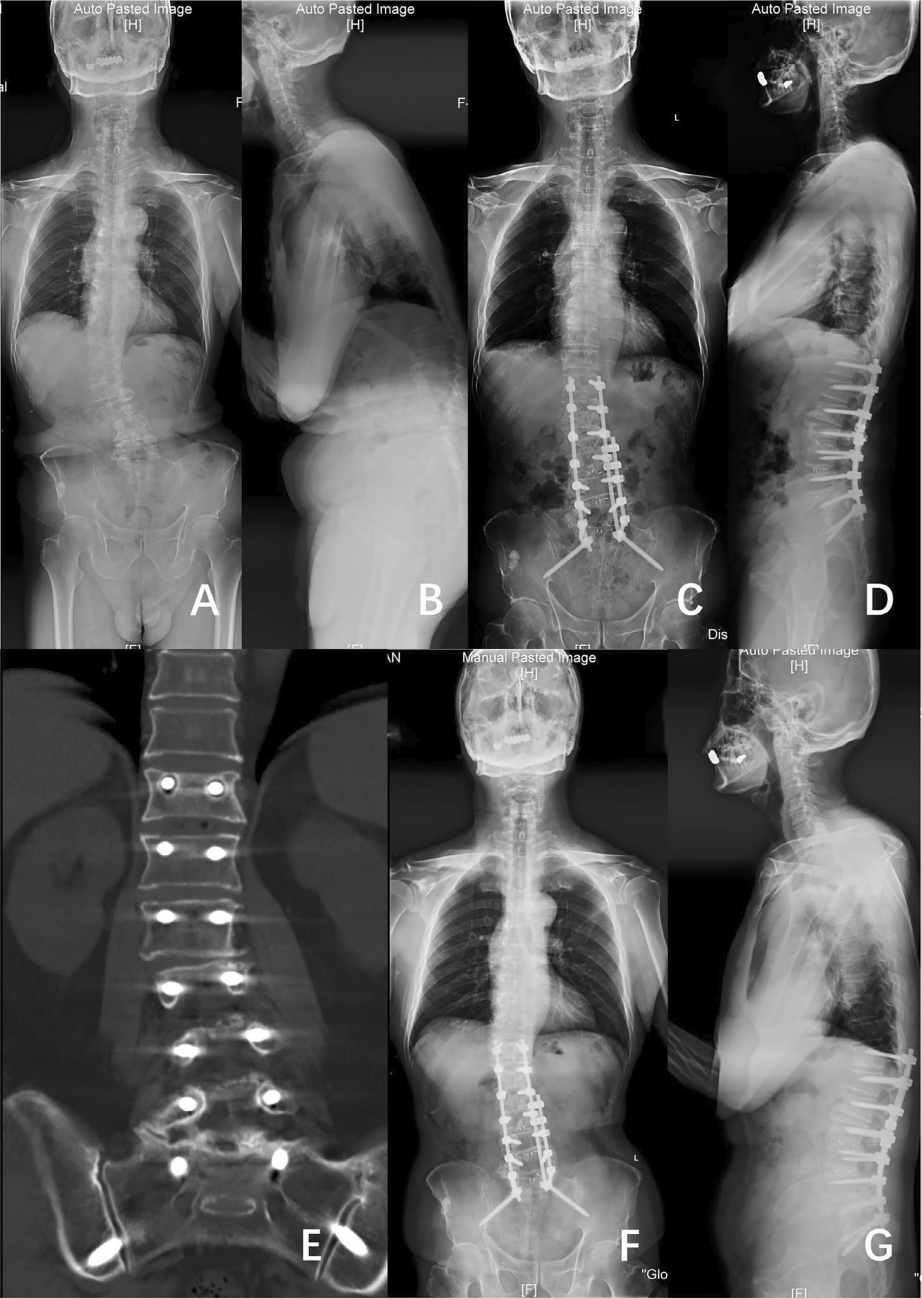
Case example of a 74‐year‐old male with DLS who underwent surgical correction consisting of T12‐S2AI posterior surgery. (A and B) Full‐length standing posteroanterior and lateral radiographs before operation (Cobb 18.6°, CDB 15.39 mm, PI 49.1°, PT 35.3°, SS 12.7°, TK 21.4°, LL 5.5°, SVA 173.56 mm). (C and D) Posteroanterior and lateral standing radiographs 1 year after operation (Cobb 5.5°, CDB 13.22 mm, PI 44.6°, PT 25.0°, SS 20.7°, TK 33.0°, LL 29.6°, SVA 15.82 mm). (E) T12 screw loosening was found 1 year after surgery by CT. (F and G) Posteroanterior and lateral standing radiographs 2 years after operation (Cobb 7.5°, CDB 10.06 mm, PI 47.7°, PT 28.4°, SS 15.6°, TK 29.9°, LL 26.1°, SVA 76.16 mm).
